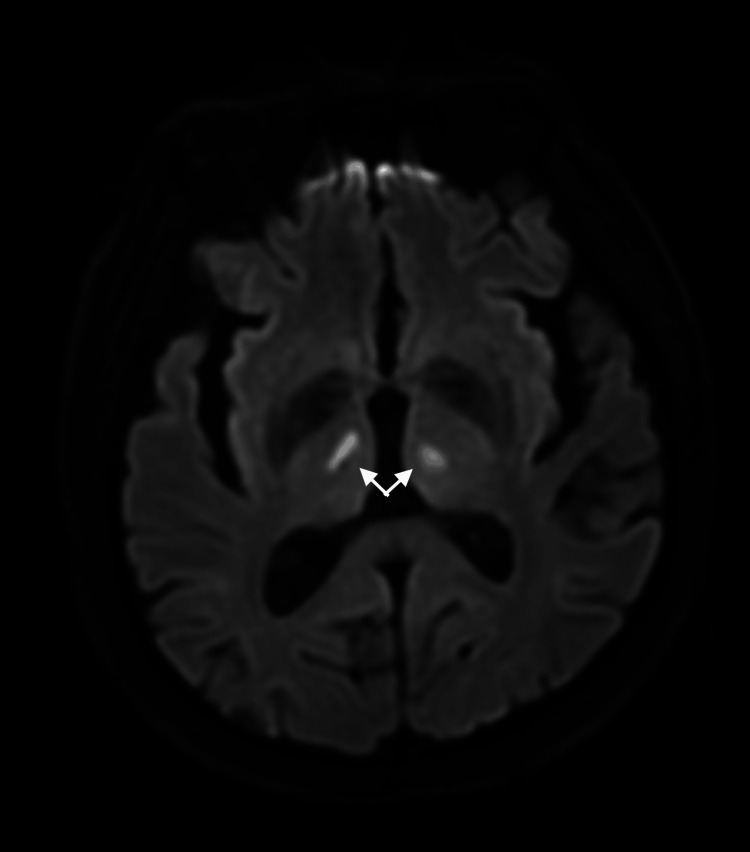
MRI-DWI sequence demonstrating bilateral thalamic ischemic strokes, indicated by white arrows. MRI-DWI: Magnetic resonance imaging-diffusion-weighted imaging
